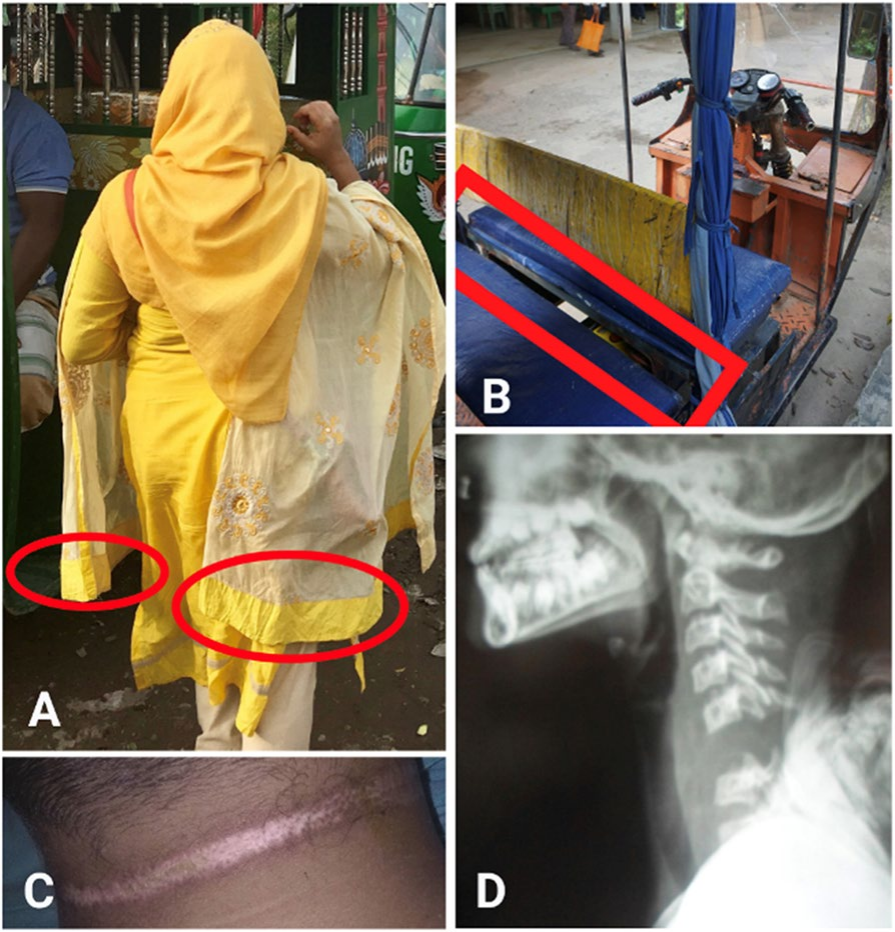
Mechanism of scarf injury and clinical presentation. (A) Visual presentation of the traditional scarf worn by a female. The red circles visualize the ends of the scarf that can slide into the gap in the easy bike and cause strangulation (B) Visualization of the Easy Bike. A red box delineates the gap between the passenger and driver seats where the scarf is caught and pulled into the driveshaft. (C) Ligature mark, present in 90% of scarf injury patients, from the forceful pull. (D) X-ray of the head and neck. A case with complete lesion (i.e., severing of the spinal cord) is pictured
